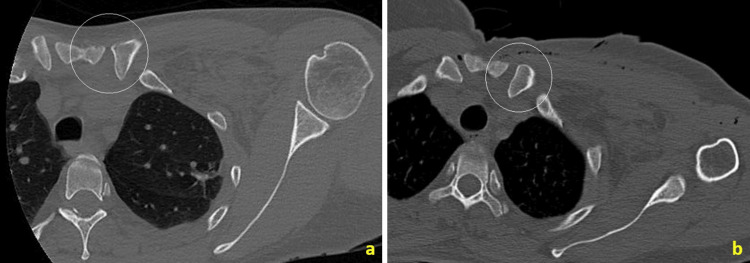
Axial cuts of the computed tomography scan in bone window settings at the level of the sternoclavicular (SC) joint in the preoperative (a) and immediate postoperative (b) phases. The left SC joint is highlighted by the white circles.
